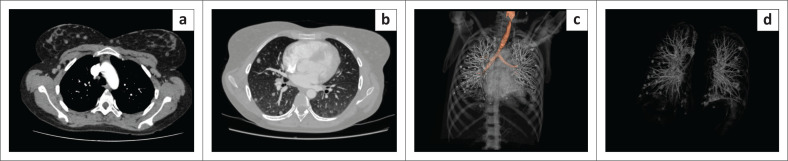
Axial CT chest images (a) and (b) and volume rendered (VR) reconstructed images (nodule analysis application) of the chest (c) and lungs (d) display numerous well-defined nodular lesions bilaterally as well as in the overlying chest wall tissues and breasts.
